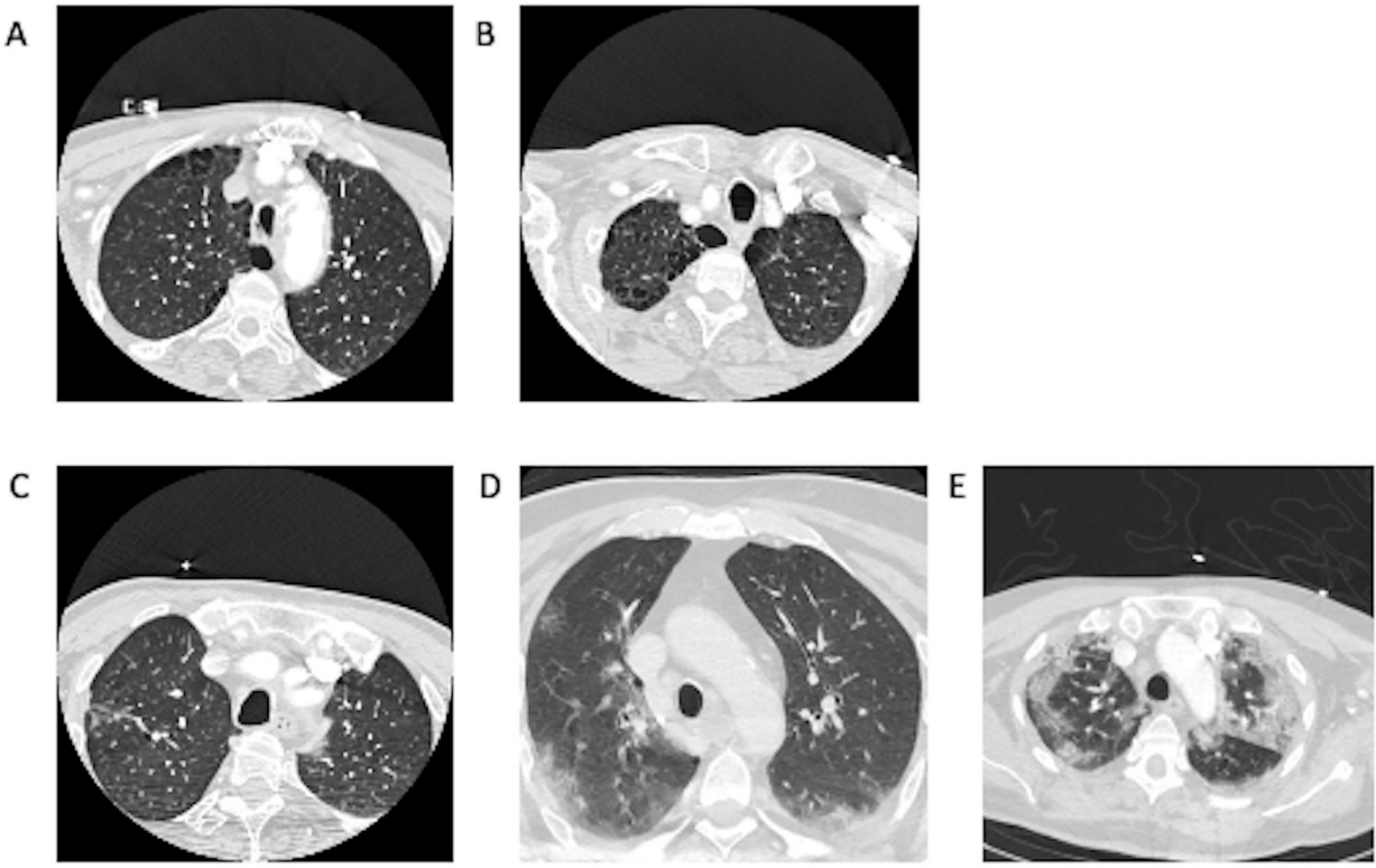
Examples for each COVID-19 Reporting and Data System (CO-RADS) score as evaluated in neck computed tomography angiography source image.
